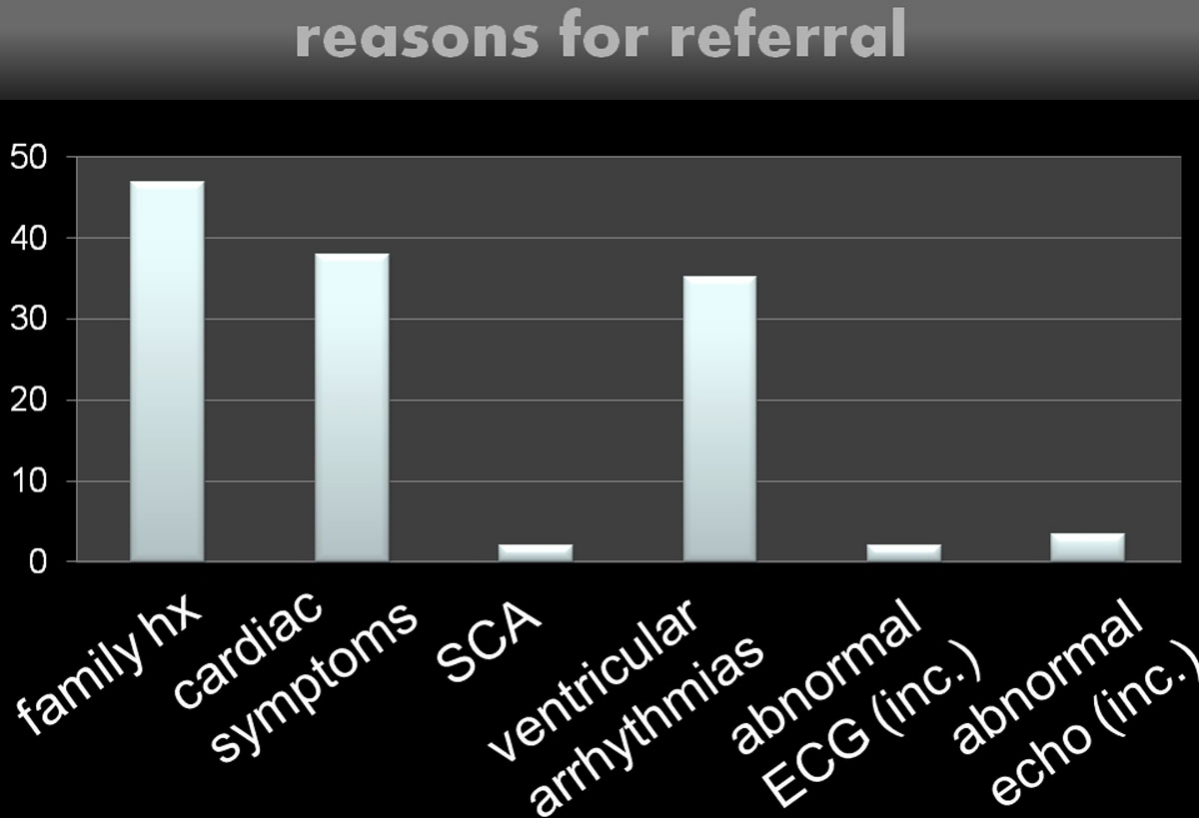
Reasons for referral for MRI to assess for signs of ARVC
